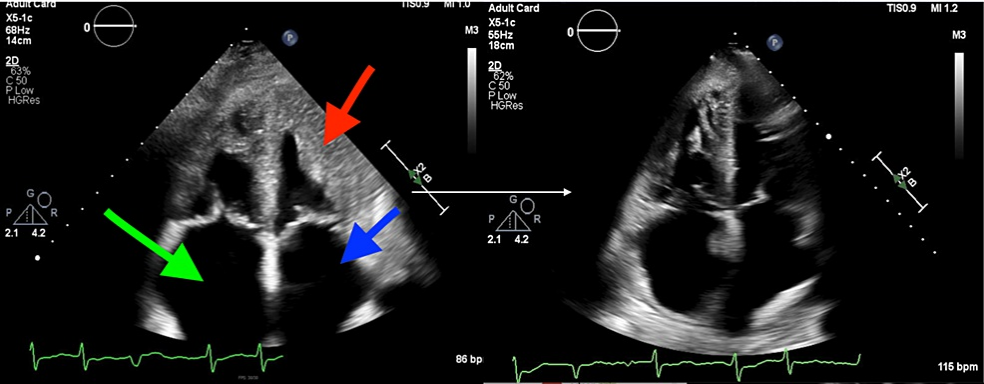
Transthoracic Echocardiogram A transthoracic echocardiogram showed concentric remodeling of the left ventricle (red arrow). The left atrium is mildly dilated (red arrow) and the right atrium (green arrow) is severely dilated.
